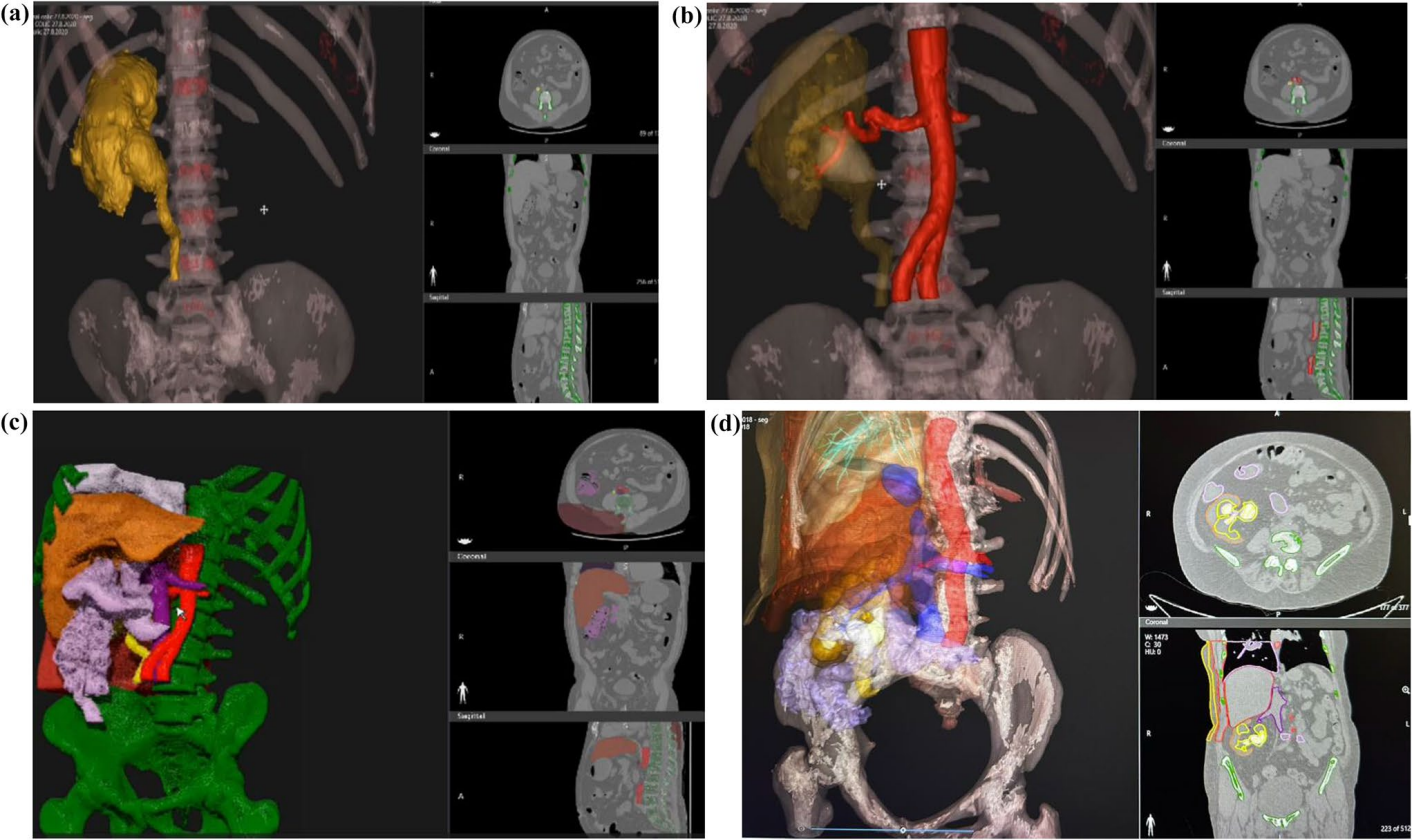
The use of axial, coronal and sagittal CT planes were applied throughout the construction of the model. The relationship between the 2D model (from the right) and the 3D model (from the left) can be observed. Each picture demonstrates a different opacity of the presented organs. a The construction of the skeletal framework and the positioning of the kidney, renal pelvis and proximal ureter in space is seen. b Next, calculating the location of the stone in the renal pelvis (painted in white), and the positioning of blood vessels is seen (aorta, renal artery and branches). Note, the construction of the IVC, renal vein and branches is not demonstrated. c The positioning of adjacent organs to the right kidney; the liver (in brown), intestines (hepatic flexure, in purple), IVC (dark purple), aorta (red), right lung (in purple), and ureter (in yellow). d A model for a right staghorn stone. The staghorn stone painted white, and the collecting system painted yellow. In addition, soft tissues, including muscles and skin are also presented. CT: computed tomography, 2D: two-dimensional, 3D: three-dimensional IVC; inferior vena cava
